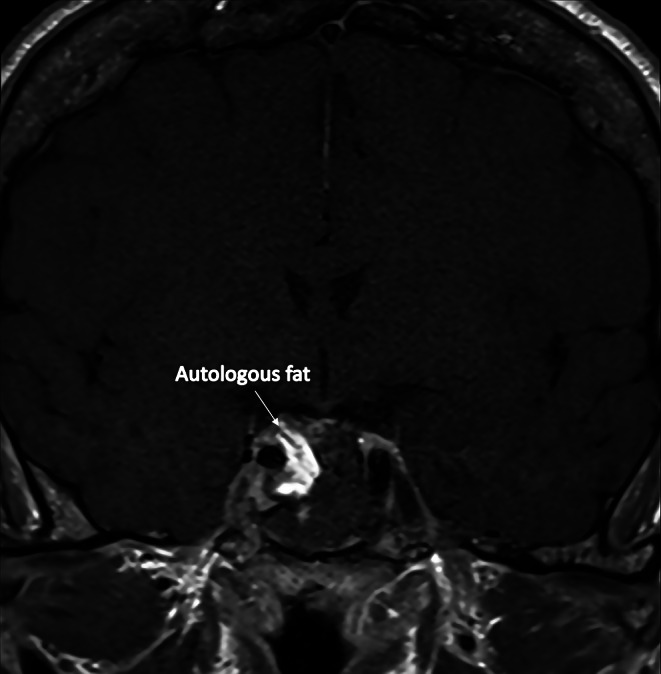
Post-operative T1W coronal MR image showing the placement of autologous fat in the resection lodge covering the carotid artery
